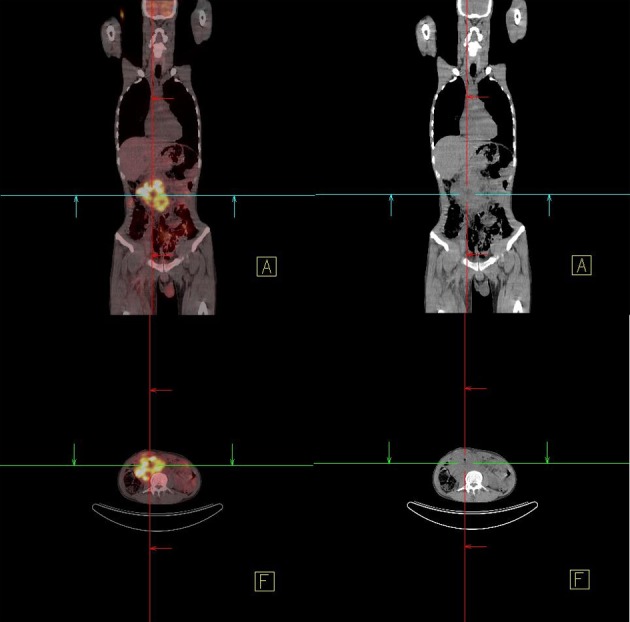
In PET-CT, a retroperitoneal mass.
